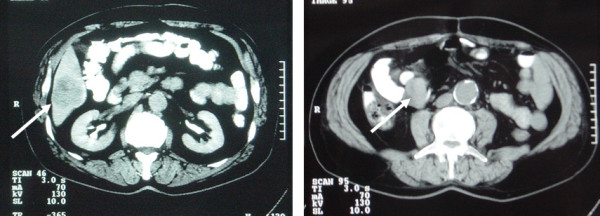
A: CT scan of the abdomen revealing, A: a 5 × 5.2 cm liver mass (white arrow) located between segments V and VI and B: a 4 × 3.8 cm soft tissue mass (white arrow) in the right abdomen between bowel loops.
